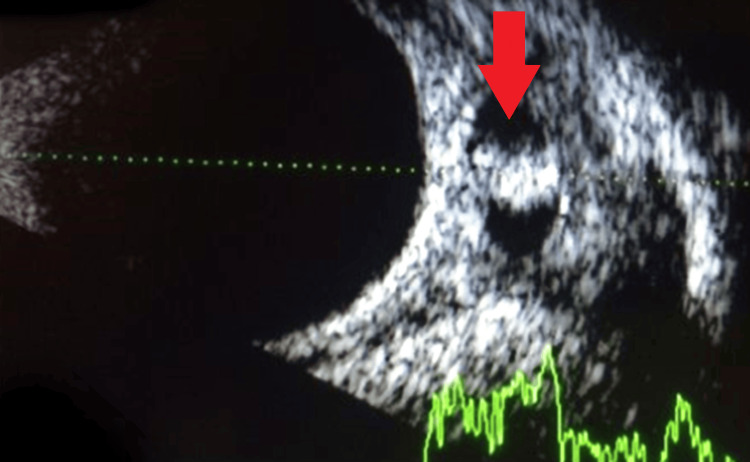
B-scan ultrasonography B-scan: brightness scan Image credit: Dr. Nivesh Gupta
